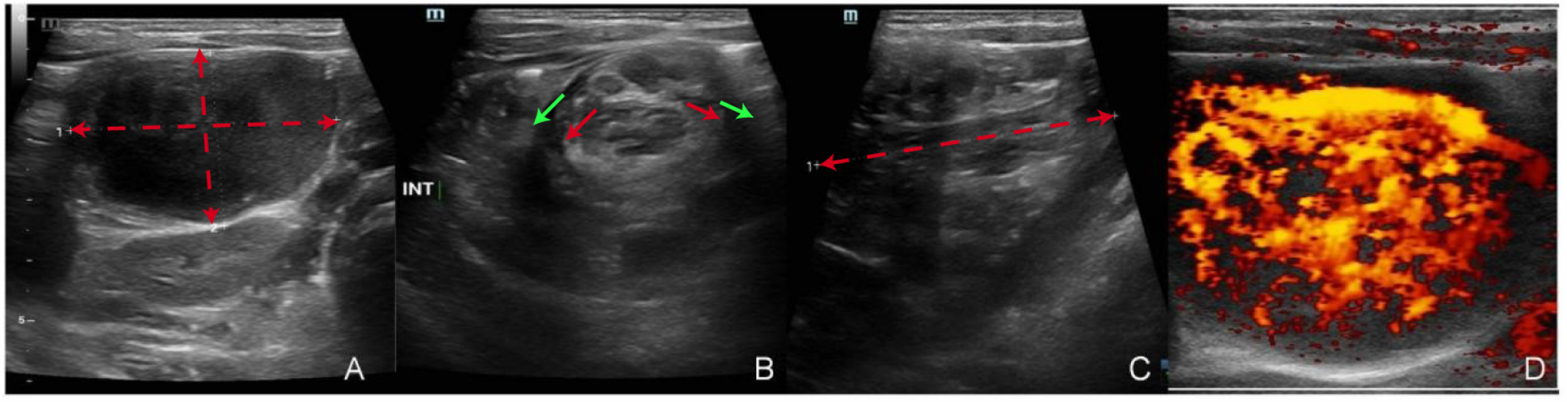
Abdominal ultrasound findings. (A) Hypoechoic mass detected in the abdominal cavity, with red dashed lines outlining its dimensions (5.7 × 3.3 × 2.8 cm). (B) Intussusception displaying distinct inner and outer layers of the intestinal wall, highlighted by red arrows (inner layers) and green arrows (outer layers). (C) Longitudinal view of the intussusception, with the red dashed line indicating the longitudinal axis. (D) Doppler imaging revealing a hypervascular mass with abundant blood supply.
